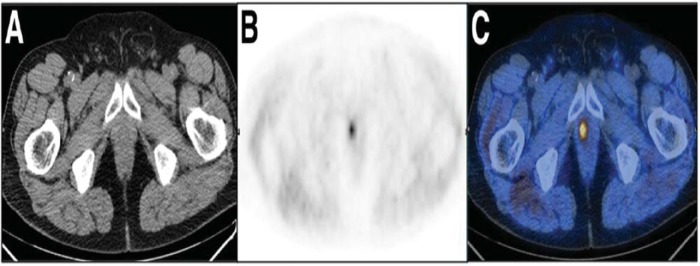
A 68-year-old man with previously diagnosed prostate cancer (Gleason score of 8) who was treated with definitive radical prostatectomy and presented with biochemical relapse (PSA, 0.85 ng/mL; PSAdt, 7.4 m; PSAvel, 0.94 ng/mL/y). 11C-choline PET/CT (A, CT; B, PET; C, PET/CT) revealed locally recurrent disease in the surgical bed. CT, computed tomography; PET, positron emission tomography; PSA, prostrate-specific antigen; PSAdt, PSA doubling time; PSAvel, PSA velocity. [Reproduced with permission from Castellucci et al (21).]
